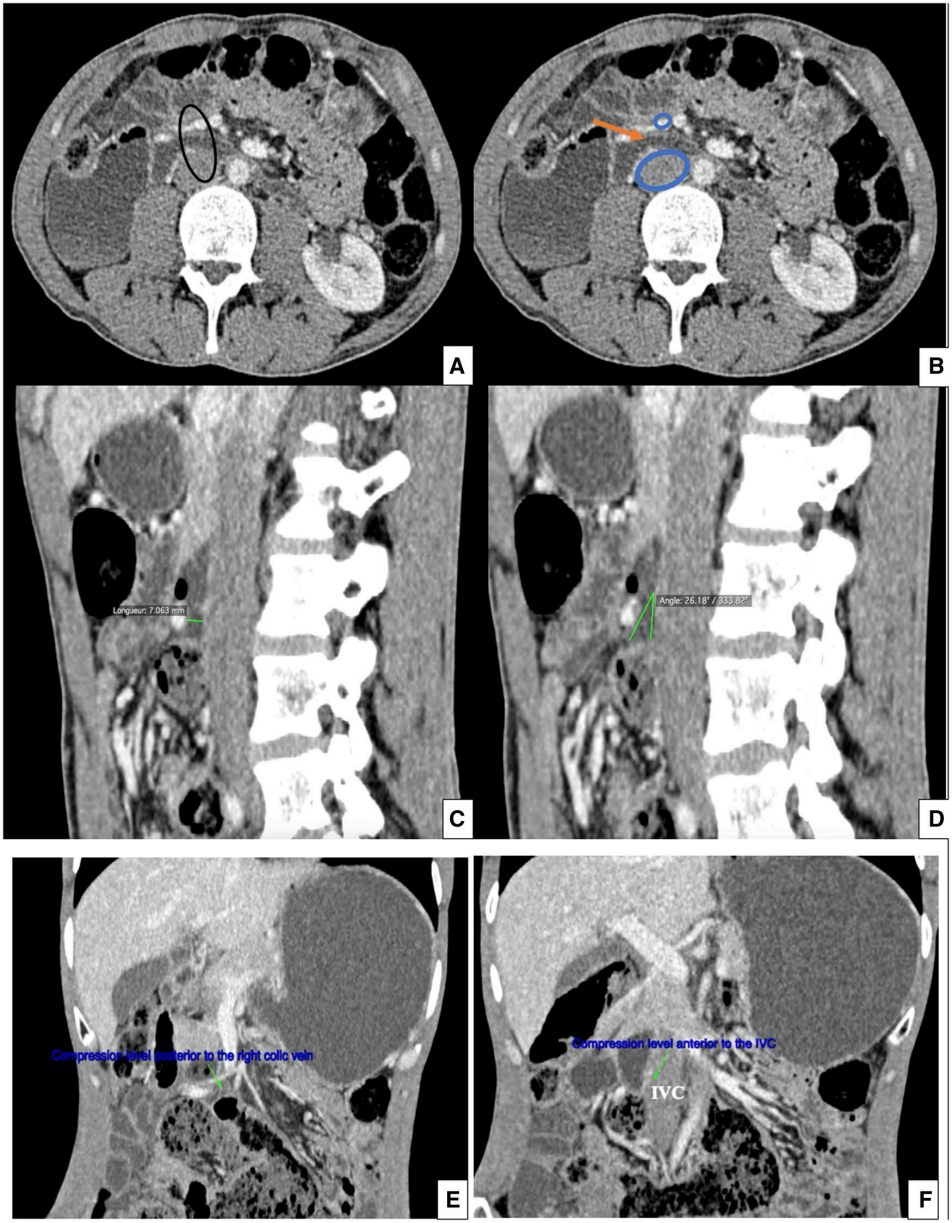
Axial (A, B), sagittal (C, D), and coronal (E, F) CT-enterography show the first level
